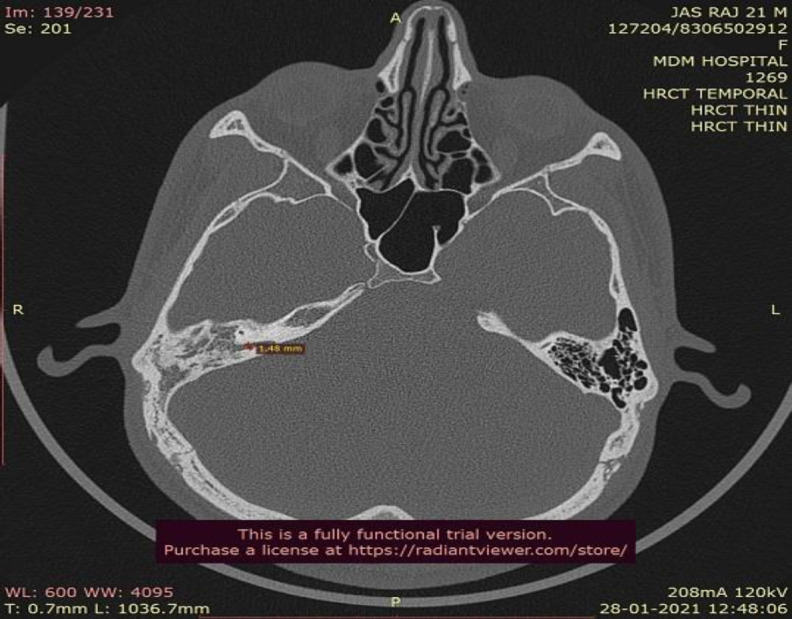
HRCT Temporal Bone scan showing enlarged petromastoid (subarcuate) canal
